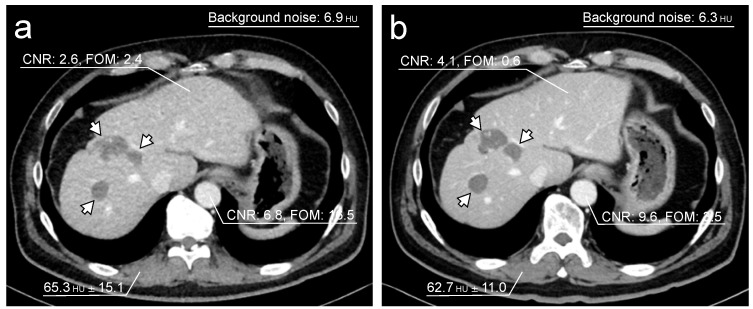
Representative single-phase enhanced CT images. Both images were acquired on separate occasions from a 62-year-old male who had liver metastases after colon cancer surgery. The tin-filtered 100 kV (TF100kV) protocol (a) shows lower CNR and higher FOM than the standard 120 kV (ST120kV) protocol (b). Liver metastases are detectable in both protocols (arrows).
